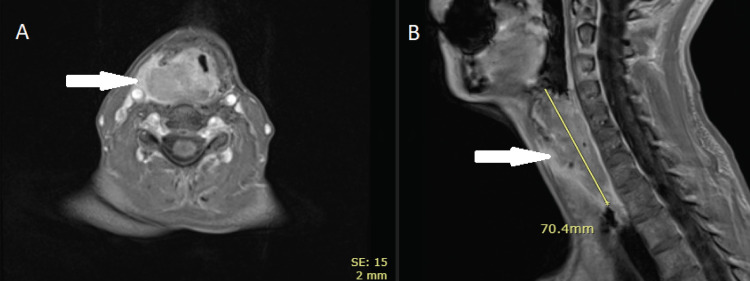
Pre-treatment magnetic resonance imaging performed before starting the chemoradiation Axial (A) and sagittal (B) T1-weighted MRI show tumors growing from the esophagus, invading the larynx.
